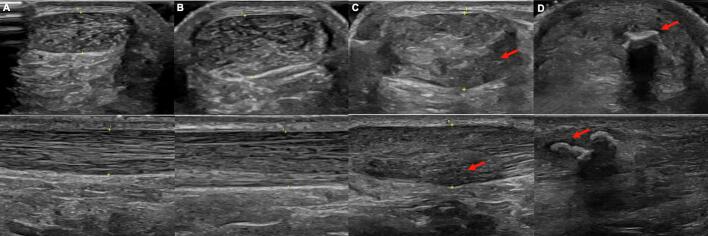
Representative ultrasonographic images of normal and abnormal Achilles tendons. The upper and lower panels represent short-axis and long-axis views, respectively. (A) Normal Achilles tendon, (B) Achilles tendon thickening without structural abnormalities, (C) Achilles tendon showing abnormal layer structure and localized hypoechogenicity (red arrow), (D) Achilles tendon with calcification (red arrow).
